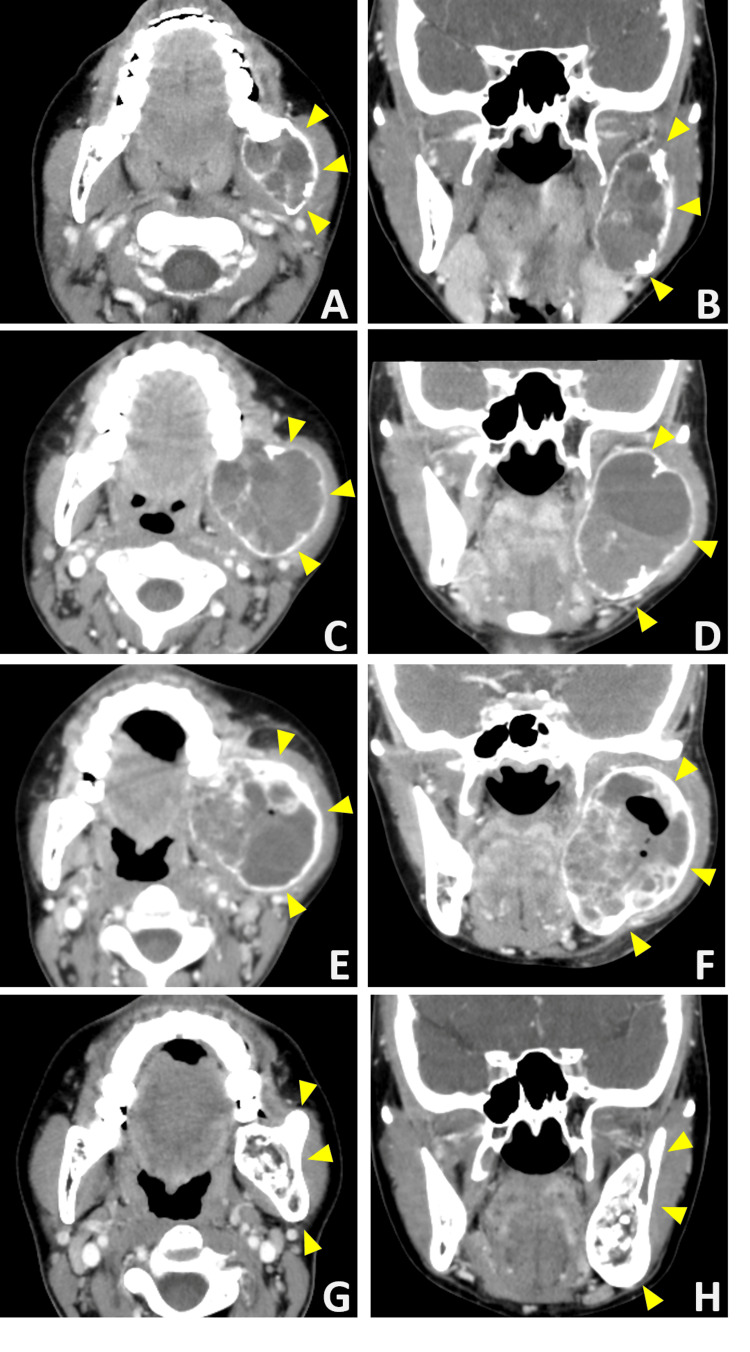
Contrast-enhanced CT images (A,B) At the initial visit, a radiolucent area measuring up to 50×30 mm was observed in the left mandibular ramus. (C,D) The lesion size had increased to a maximum of 65×40 mm before sclerotherapy. (E,F) After sclerotherapy, the lesion showed mild reduction with marginal calcification. (G,H) At 24 months post operation, the lesion had resolved, with evidence of bone regeneration. The arrows indicate the lesion and the healed mandibular ramus.
